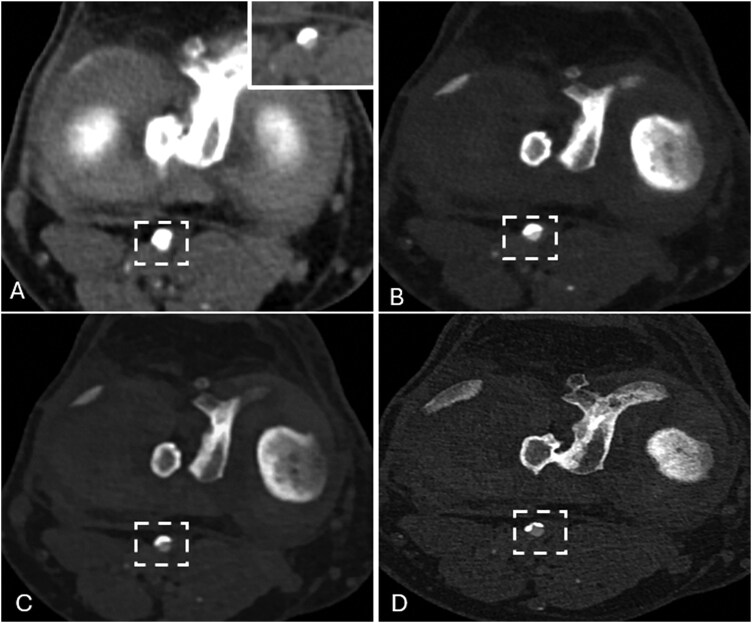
A 77-year-old male with calcified plaques in the popliteal artery underwent EID-CTA (A), demonstrating a calcific plaque with blooming artifact (white dashed box) that prevents accurate visualization of the lumen, even in a delayed phase (A inset). Same-day PCD-CT with different reconstructions shows calcium blooming artifact (white dashed box in B) with a smooth kernel (Qr48), with decreased blooming using a high-energy VMI (100 keV; white dashed box in C) even when using a smooth kernel; this is further reduced when a high-energy VMI (100 keV) is combined with a sharp, higher-resolution reconstruction kernel (Bv68; white dashed box in D). Abbreviations: Abbreviations: CTA, CT angiography; EID, energy-integrating detector; PCD, photon-counting detector; VMI, virtual mono-energetic images).
